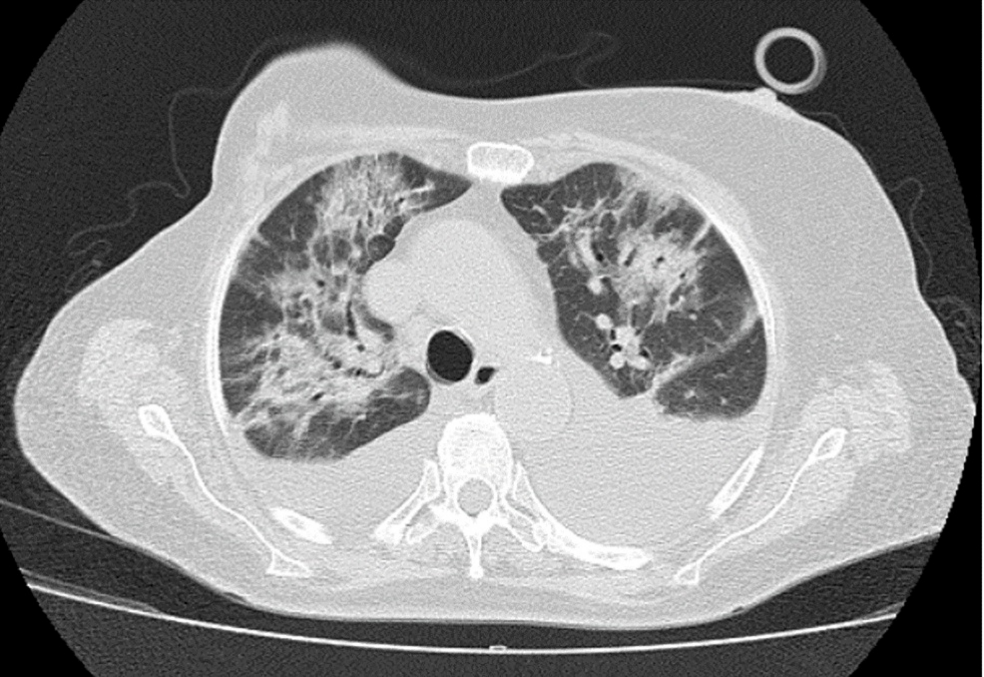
Chest-CT scan with bilateral pleural effusion and sparse ground-glass areas.
